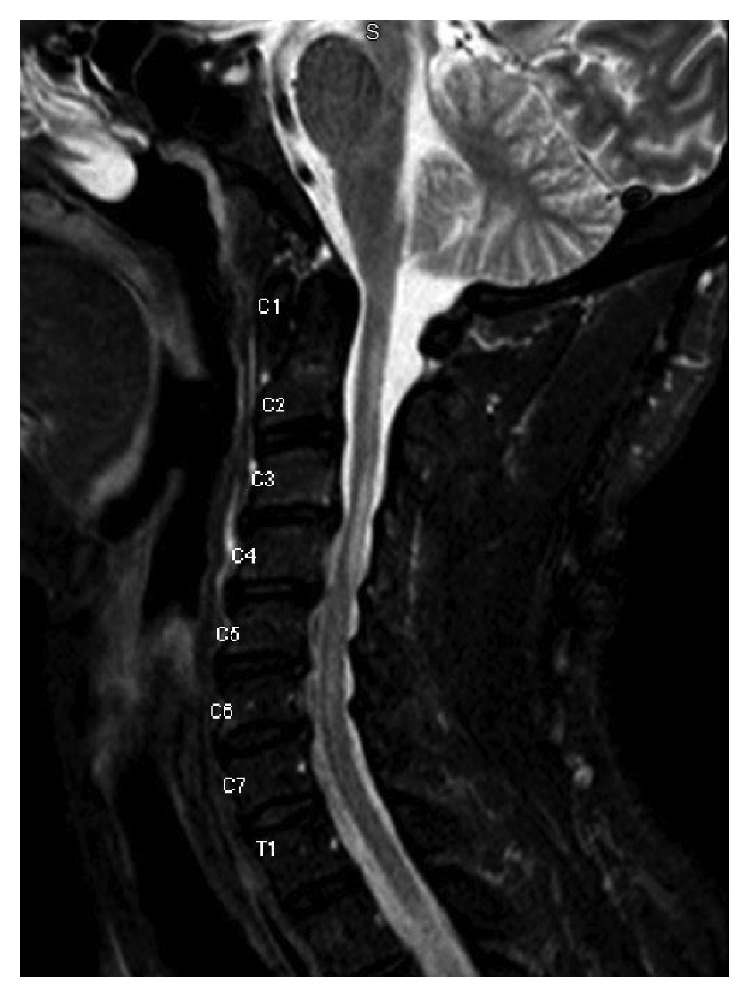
Hyperintense lesions representing demyelination from C2 to C6 on MRI C-Spine T2 FLAIR sequencing.
